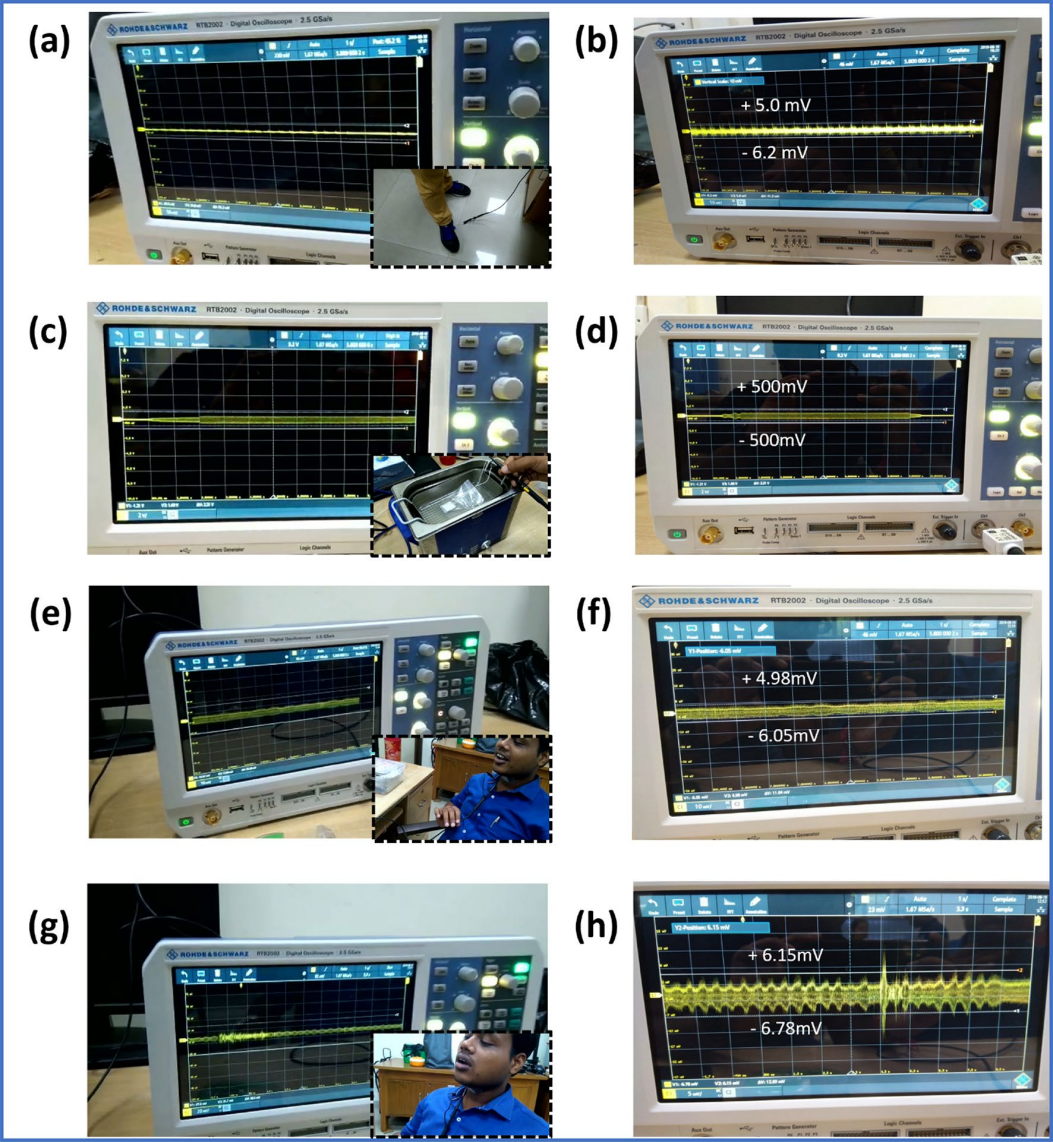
(a) Output voltage generation from the leg motion (inset shows the device attached below the shoe), (b) enlarge view of the output signal depicted in (a), (c) Output voltage generation from the ultrasonic vibration (inset shows the device placed in the ultrasonic bath), (d) enlarge view of the output signal depicted in (c), (e) output voltage generation from the vocal sound (inset shows the device attached on the throat), (f) enlarge view of the output signal depicted in (e), (g) output voltage generation from the jaws motion (inset shows the device attached on the throat), and (h) enlarge view of the output signal depicted in (g).
